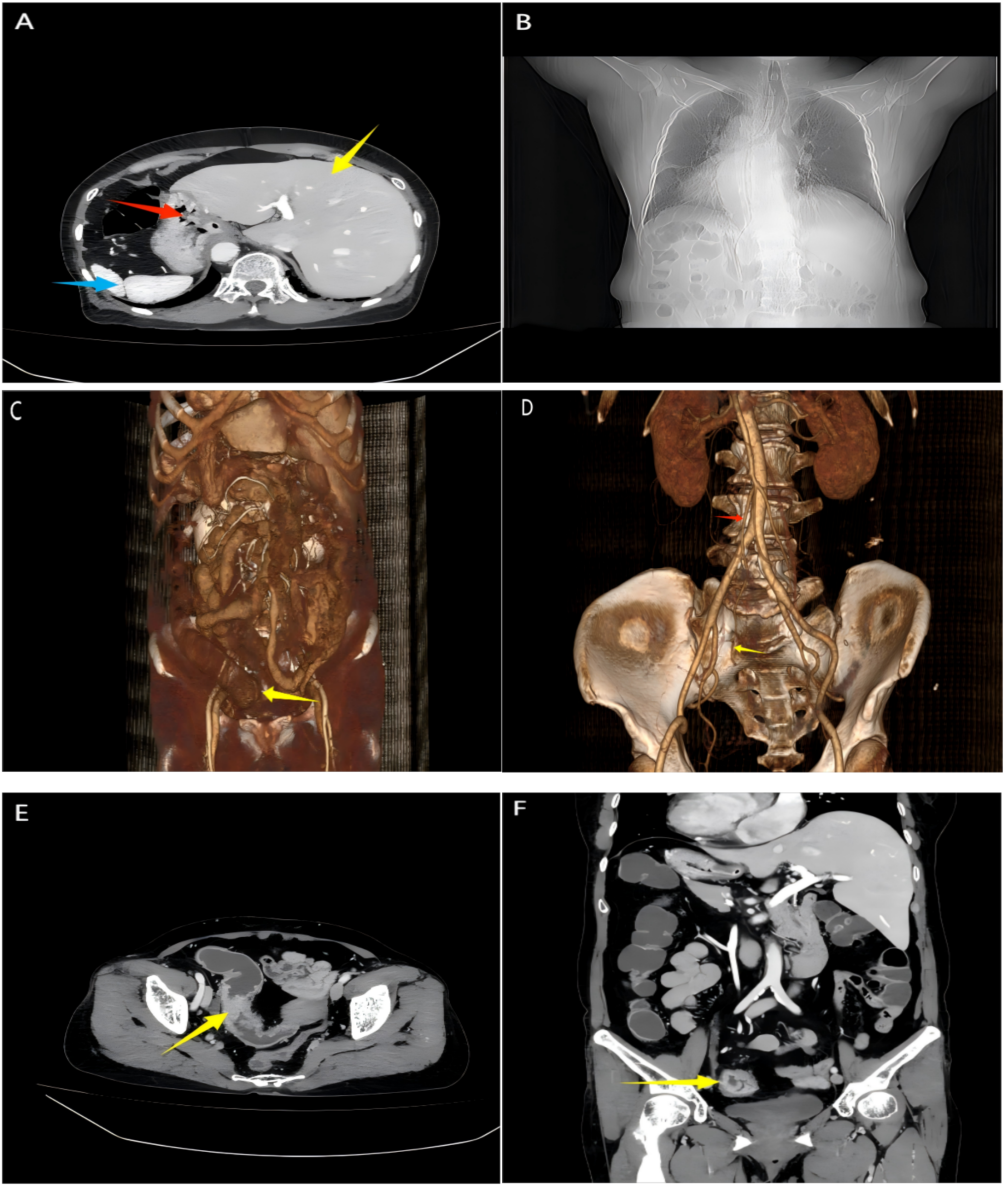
Images of computed tomography. (A) CT scan shows reversed positions of the liver (Red arrow), stomach (Red arrow), and spleen (blue arrow); (B) Chest CT shows dextrocardia; (C) 3D CT colonography shows a sigmoid colon tumor (yellow arrow). (D) The three-dimensional reconstruction images of CT angiography show the inferior mesenteric artery (red arrow) and sigmoid artery (yellow arrow); (E, F) CT scan cross-sectional/coronal images suggest a mass in the sigmoid colon.
